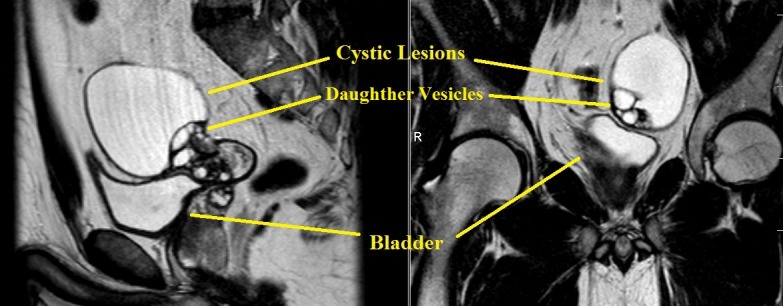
MRI aspect of the cystic lesions
